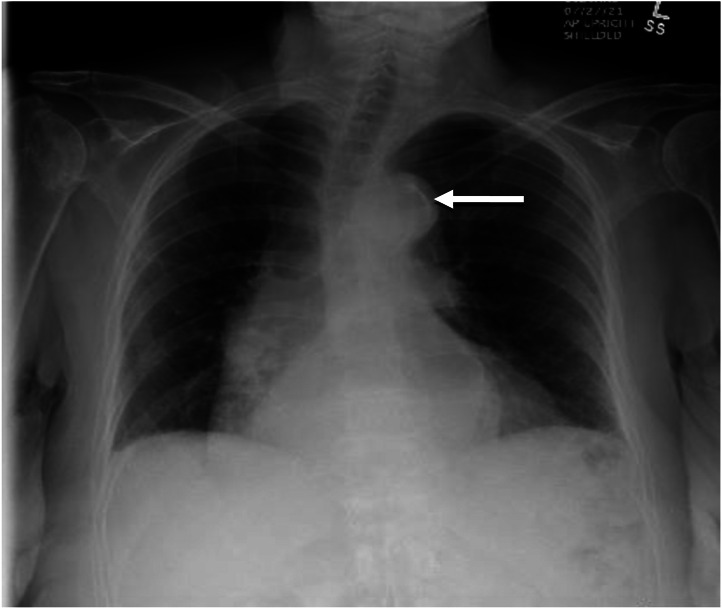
Chest radiograph showing mild left costophrenic blunting and atherosclerotic calcification of the aorta (arrow).
